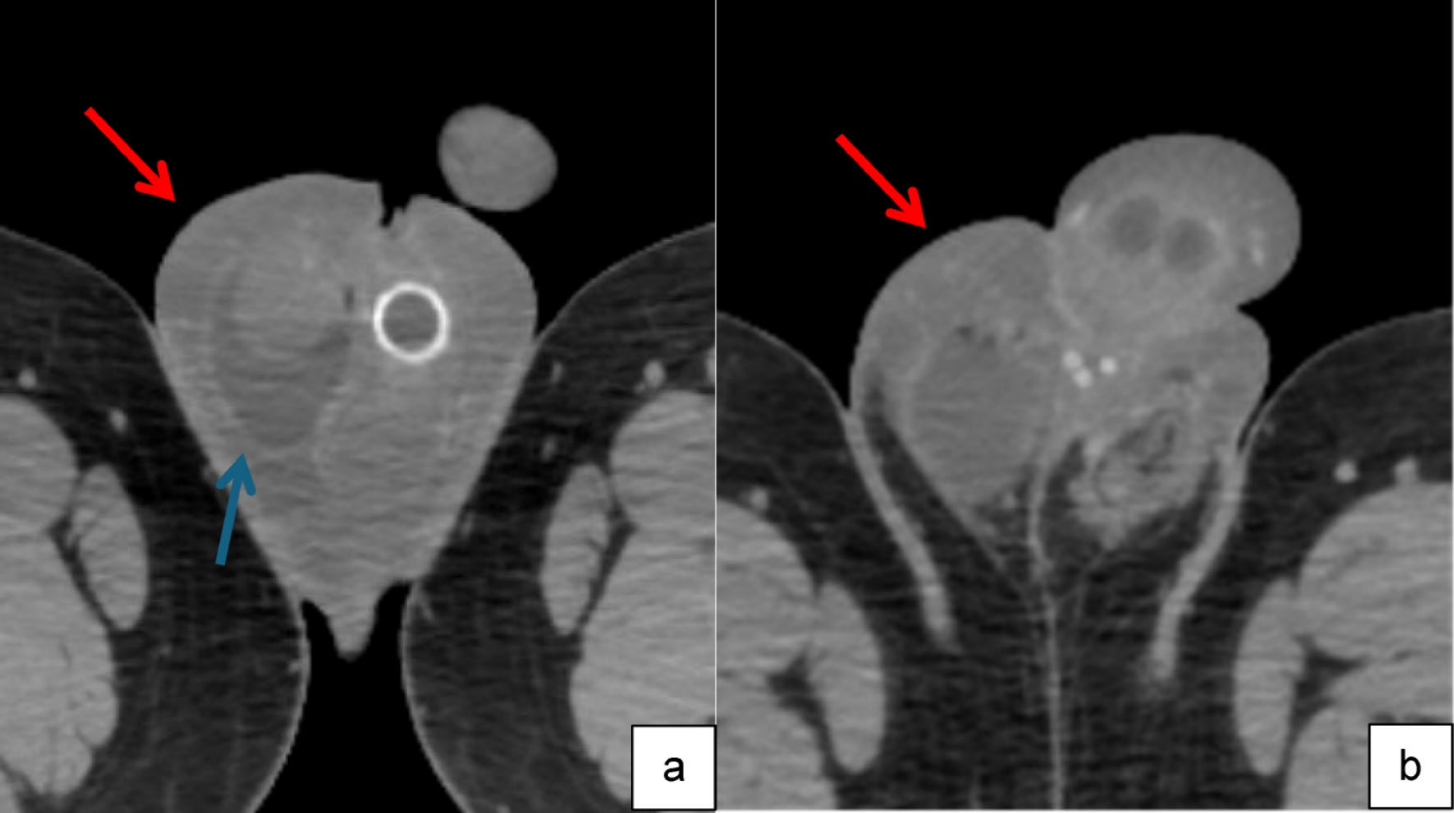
Axial images inferior (a) and superior (b) from CT pelvis with IV contrast show diffuse penoscrotal skin thickening and subcutaneous edema (red arrow) without discrete hematoma or rim-enhancing fluid collection. Small right hydrocele is incidentally noted (blue arrow). Portion of a PP pump is visible
